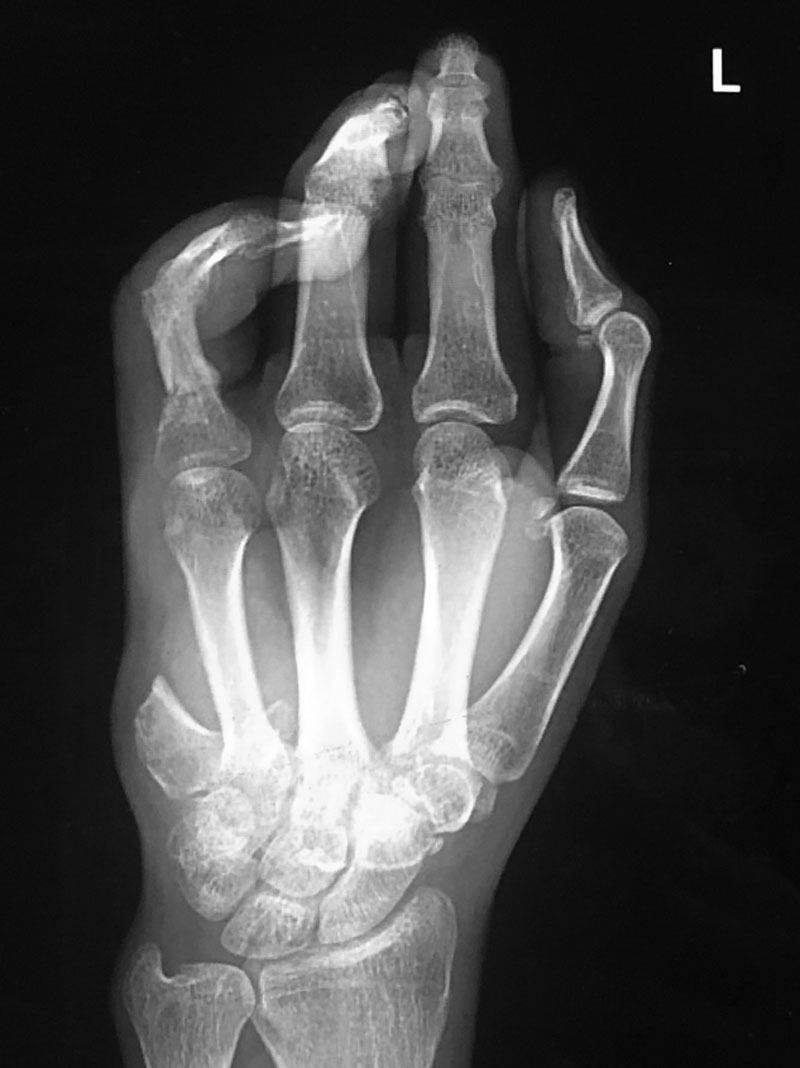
Radiograph demonstrating osteosynthesis at 7-weeks postoperatively after reconstruction of the ring finger using a small finger ulnar digital artery-based osteocutaneous fillet flap.
